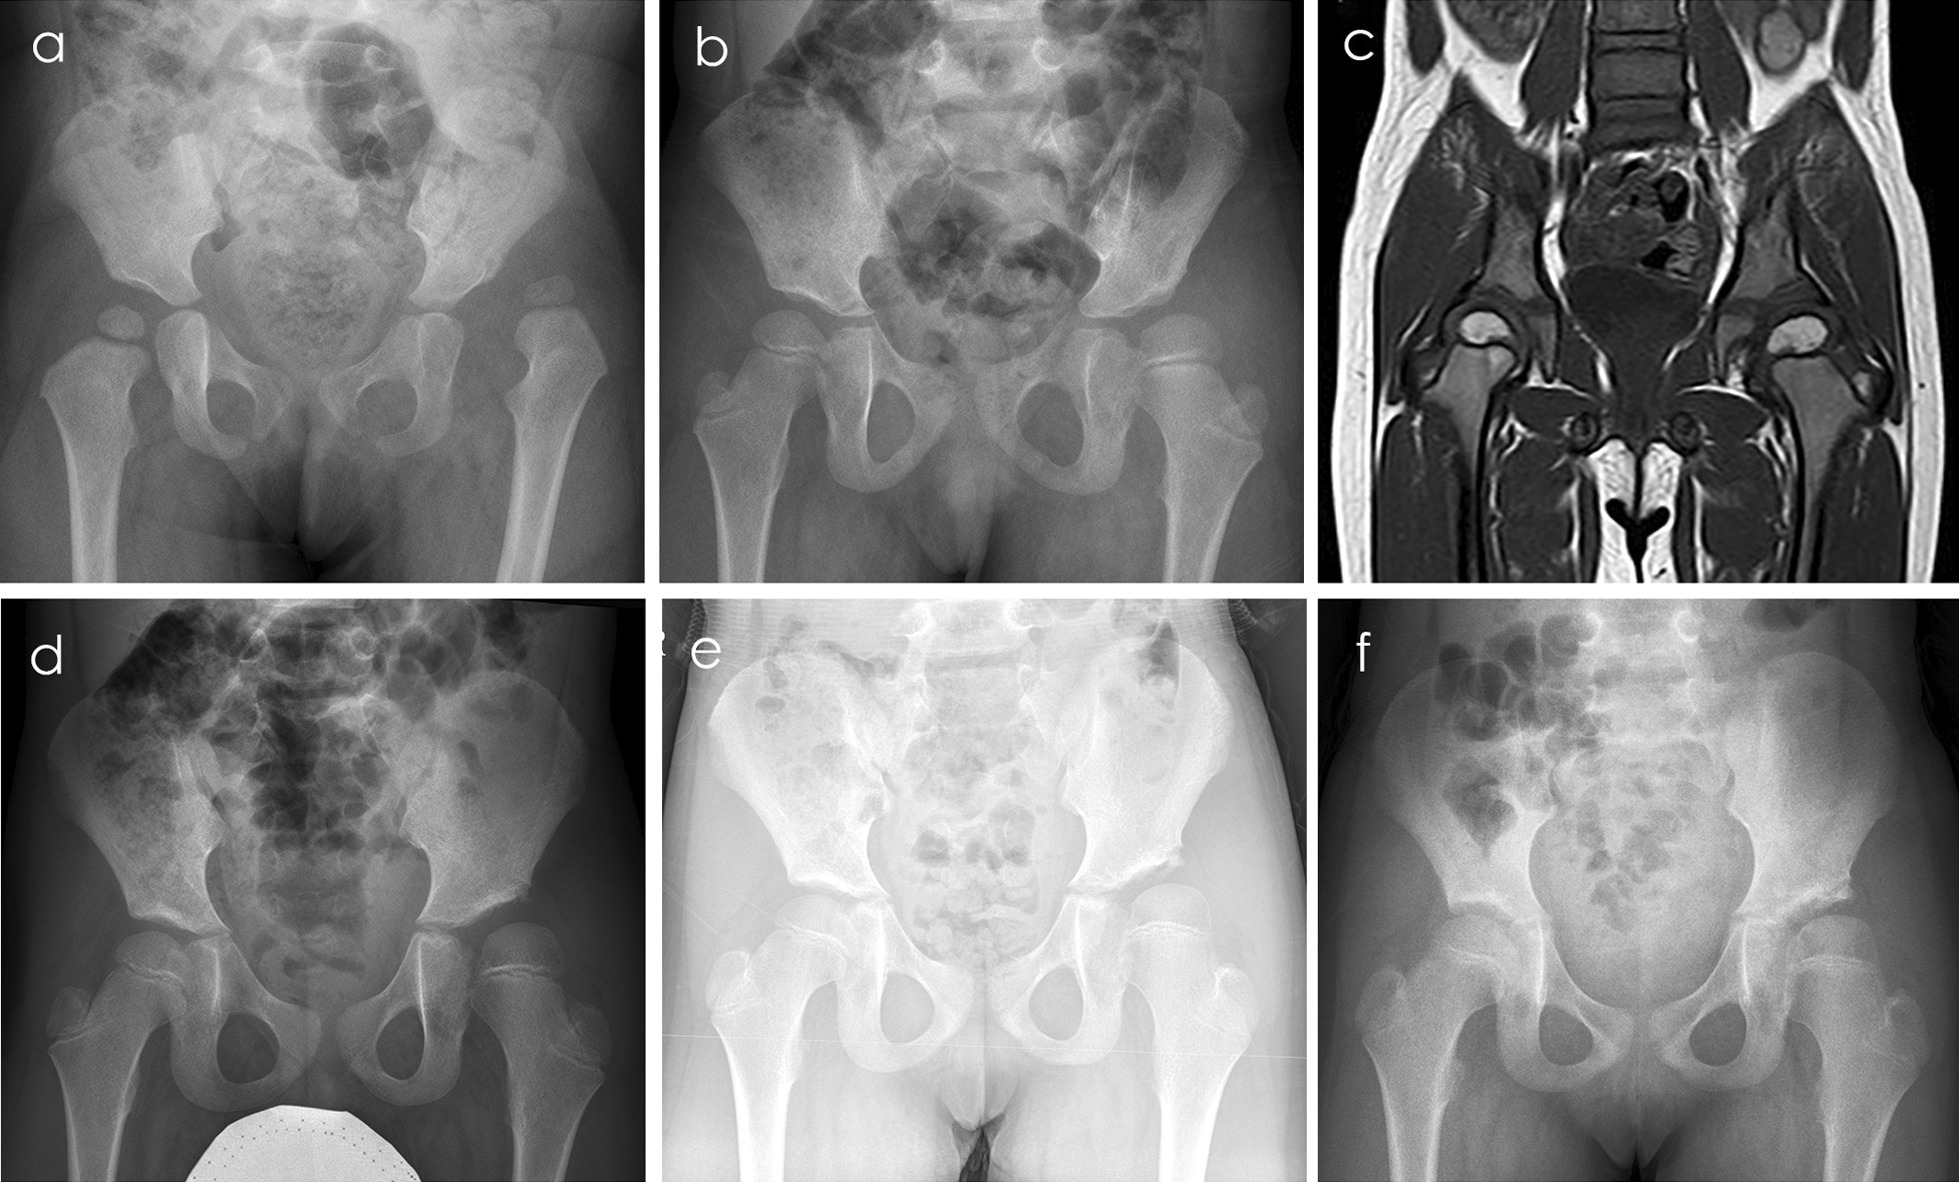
a Preoperative pelvic anteroposterior view in a 17-month-old girl on left hip dislocation with the modified Smith–Petersen approach. b, d–f Radiograph at 32, 64, 90, and 103 months after open reduction, respectively, without obvious AVN sign. f demonstrates suspicious early signs of type 2 AVN such as slightly laterally inclined proximally convex physis, medial femoral head flattening and an oval-shaped epiphysis. c MRI assessment at 32 months after open reduction
